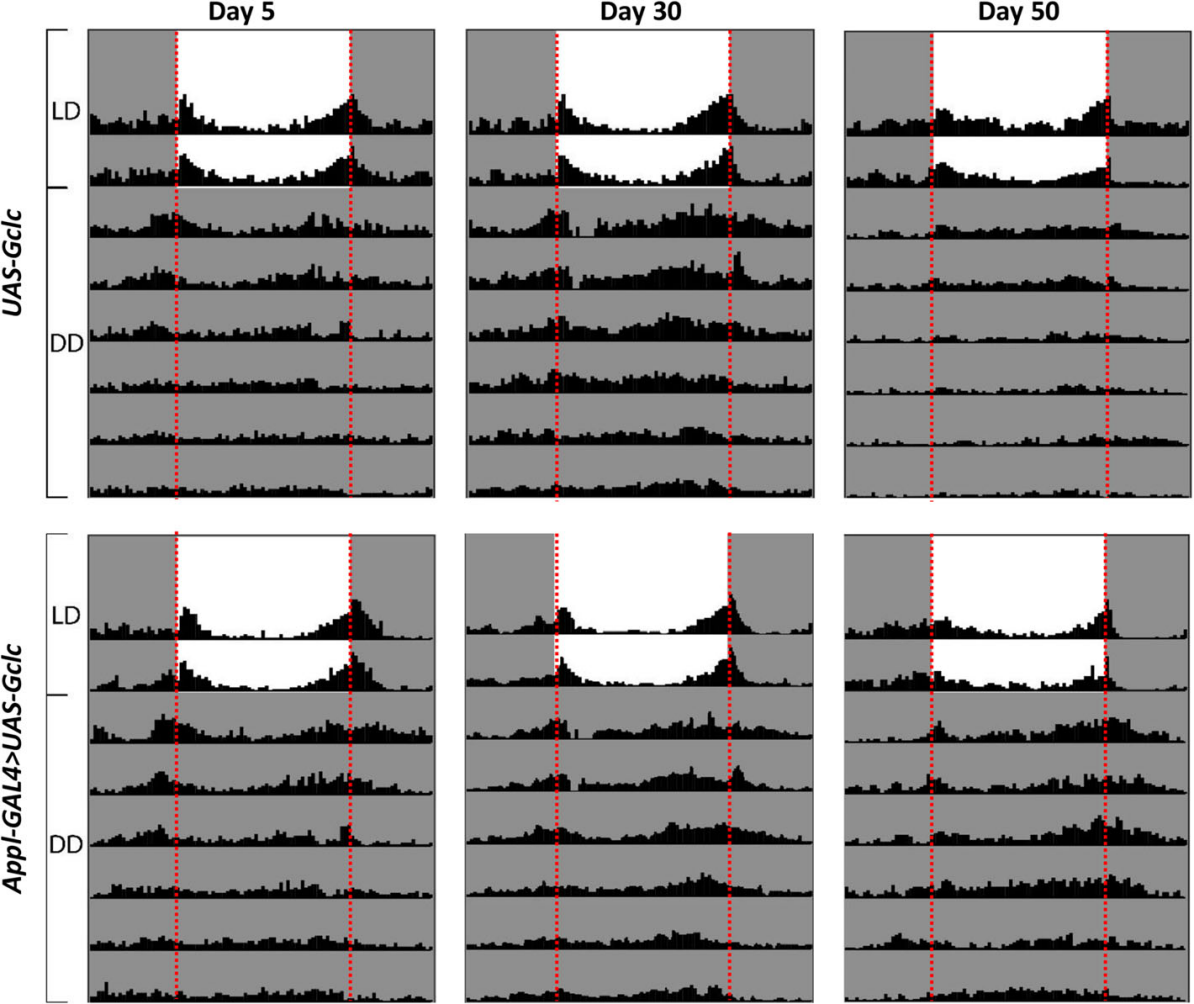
Influence of *Gclc* overexpression on age-dependent disruption of locomotor activity rhythms. Locomotor activity profiles of representative 5-, 30-, and 50-day-old males of the indicated genotypes. Flies of each age were monitored in LD (12:12) for 2-d, followed by 6-d in DD at 25 °C. Shaded areas represent periods of darkness. Vertical red dotted lines indicate time of lights-on (ZT/CT 0) and lights-off (ZT/CT 12)
